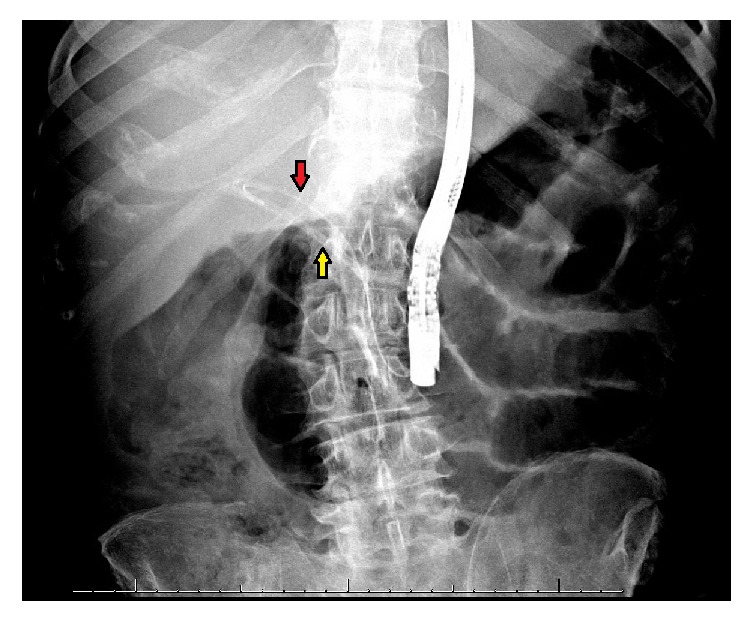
Abdominal radiograph obtained during bedside ERCP showing sphincterotome inside the common bile duct (red arrow). The migrated stent (yellow arrow) is seen with the distal tip inside the bile duct.
